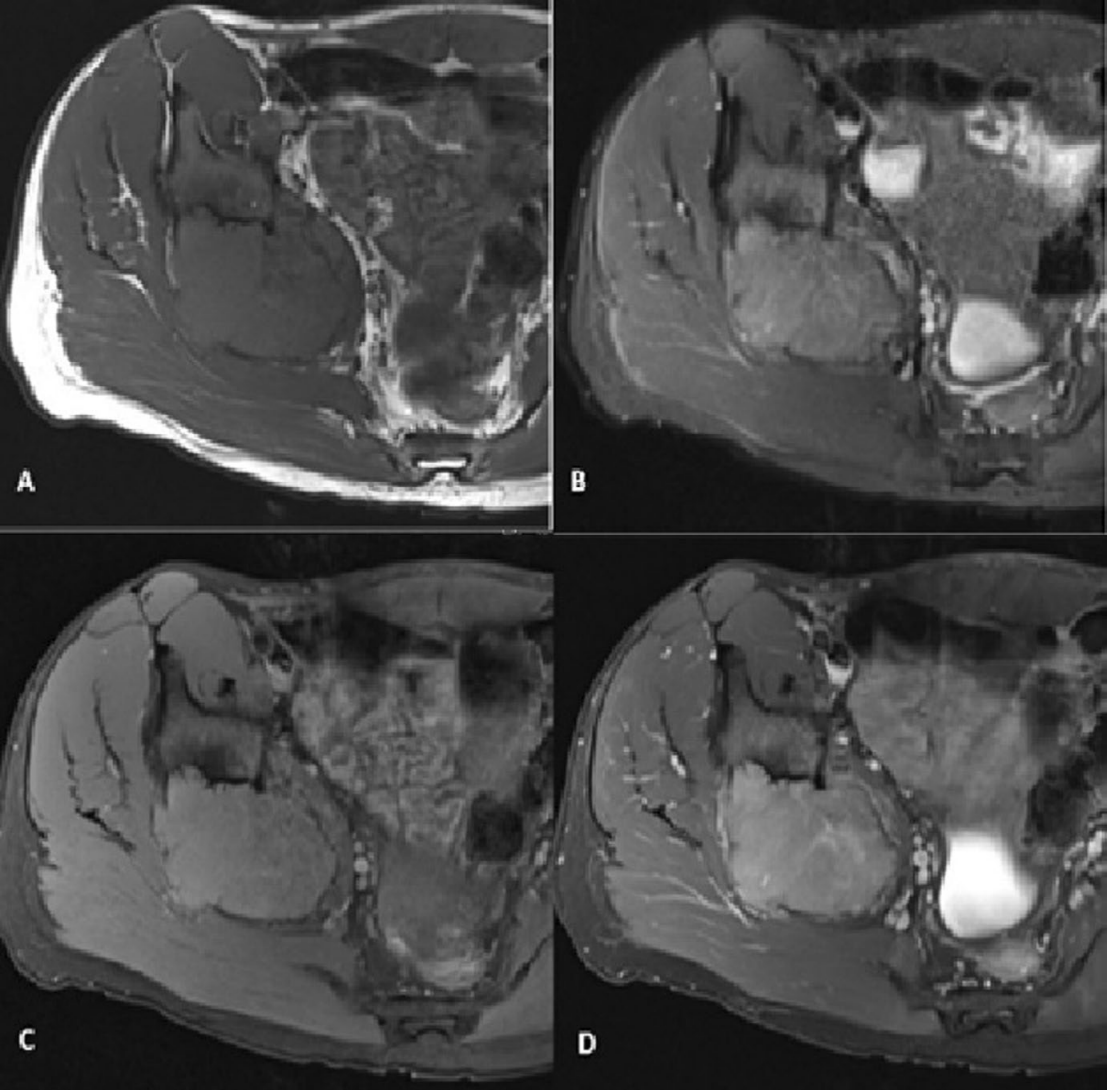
Axial MRI images of the right hip joint. (A, B) The lesion appears isointense to muscles on T1‐weighted (T1W) images and demonstrates intermediate signal intensity on STIR images. (C) Pre‐contrast T1W fat‐saturated (T1W FS) image and (D) mild diffuse enhancement on post‐contrast T1W FS images.
